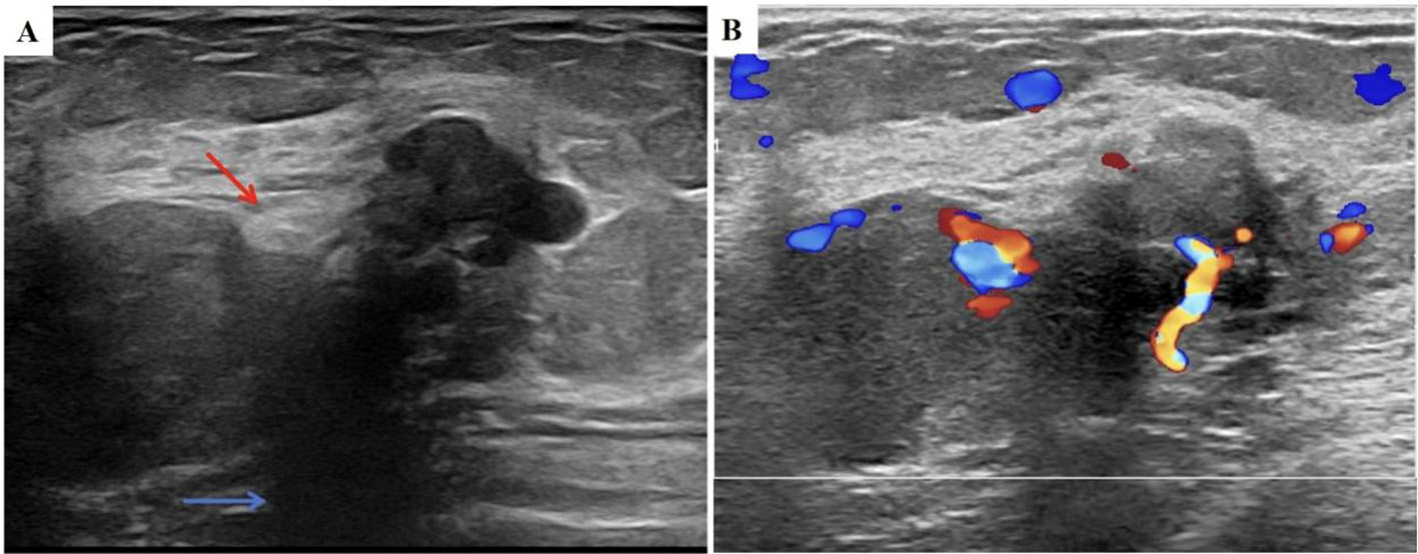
US images of the tumor. (A) US of right breast nodule (this image was taken on June 11, 2024. The calcifications is identified by the red arrow, and the acoustic shadowing is marked with the blue arrow); (B) Color Doppler image of right breast nodule (this image was taken on June 11, 2024); US, ultrasound; SWE, Shear wave elastography; SE, Strain elastography.
